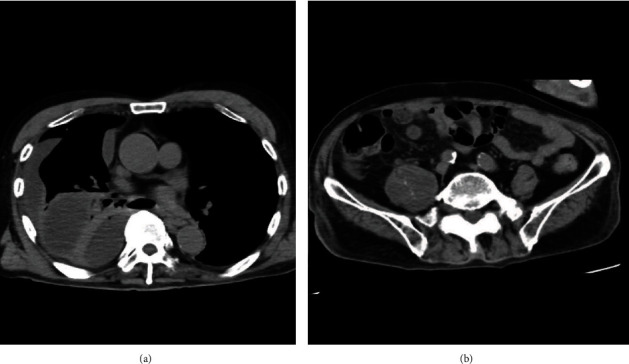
Truncal computed tomography (CT) findings on arrival. Truncal CT (axial section) suggested right multilobular empyema (a) and right iliopsoas abscess (b).
